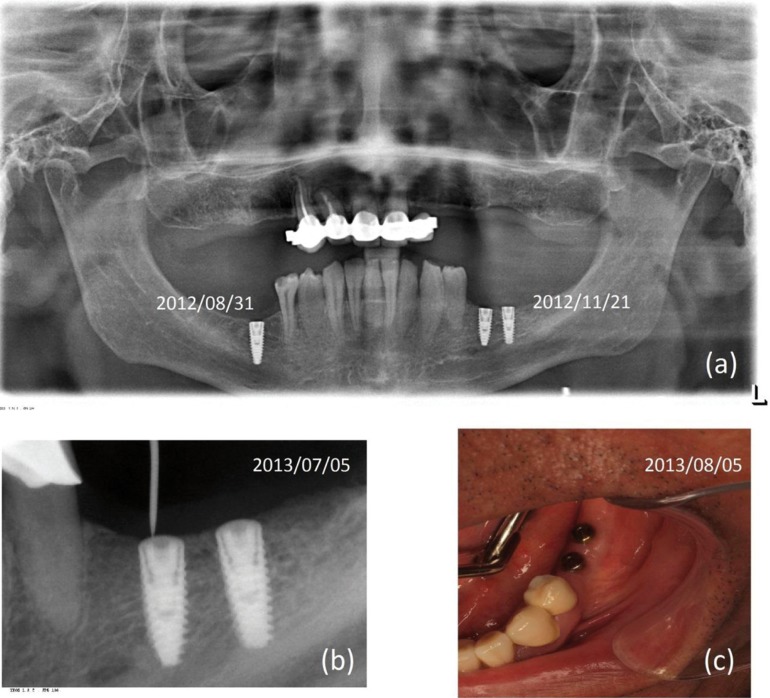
Radiographic and soft-tissue controls. (a) OPG with placement dates of implants. (b) Integrated bone implants are seen. Exploration for the placement of healing abutments. (c) Healthy soft tissues with healing abutments.
